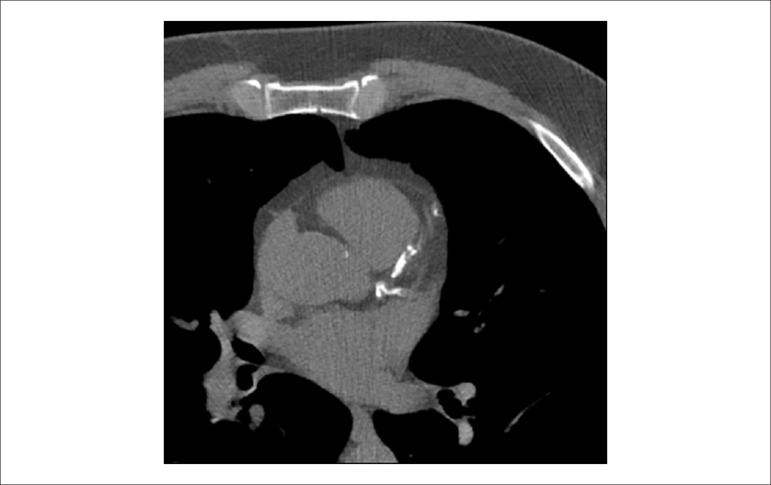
Case 4 - Angio-CT imaging shows elevated calcification index in coronary arteries.
